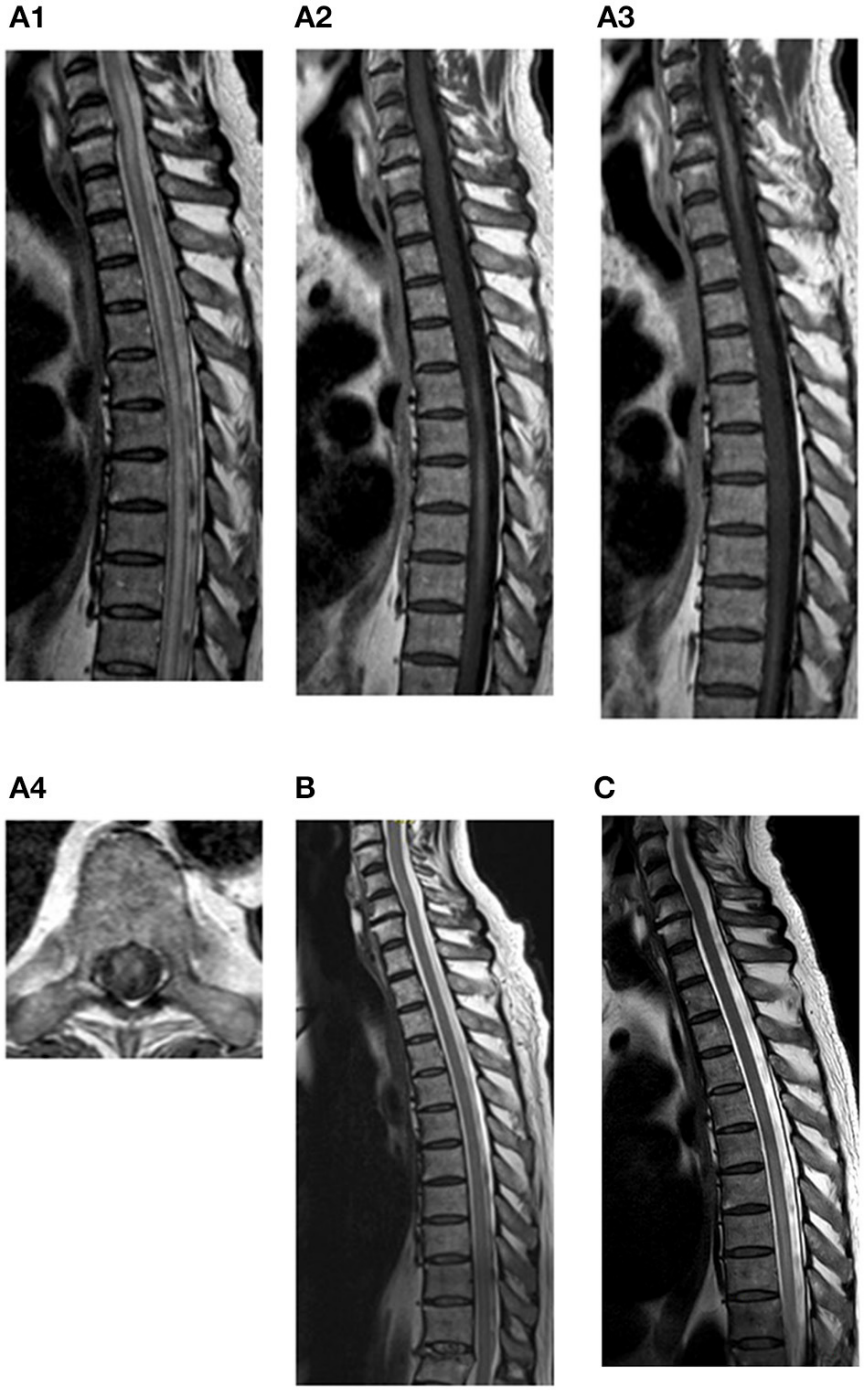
MRI progression: at time of diagnosis (A1–4), 3 days after last dose of p.o. MP (B) and 11 days after last dose of p.o. MP (C). (A1) Sagittal T2-weighted MRI of columna totalis showing extensive LETM at cervical and thoracic levels. (A2) Sagittal T1-weighted MRI of columna totalis with contrast showing contrast enhancement at levels Th5–Th9. (A3) Sagittal T1-weighted MRI with contrast showing enhancement from C4-Th1. (A4) Axial T1-weighted MRI with contrast showing anterolateral multifocal contrast enhancement at level Th9. (B) Sagittal T2-weighted MRI of columna totalis showing almost complete regression of LETM. No contrast enhancement on T1 (not shown). (C) Sagittal T2-weighted MRI of columna totalis with relapsing LETM on levels Th5–Th11. Contrast enhancement on T1 weighted MRI (not shown).
